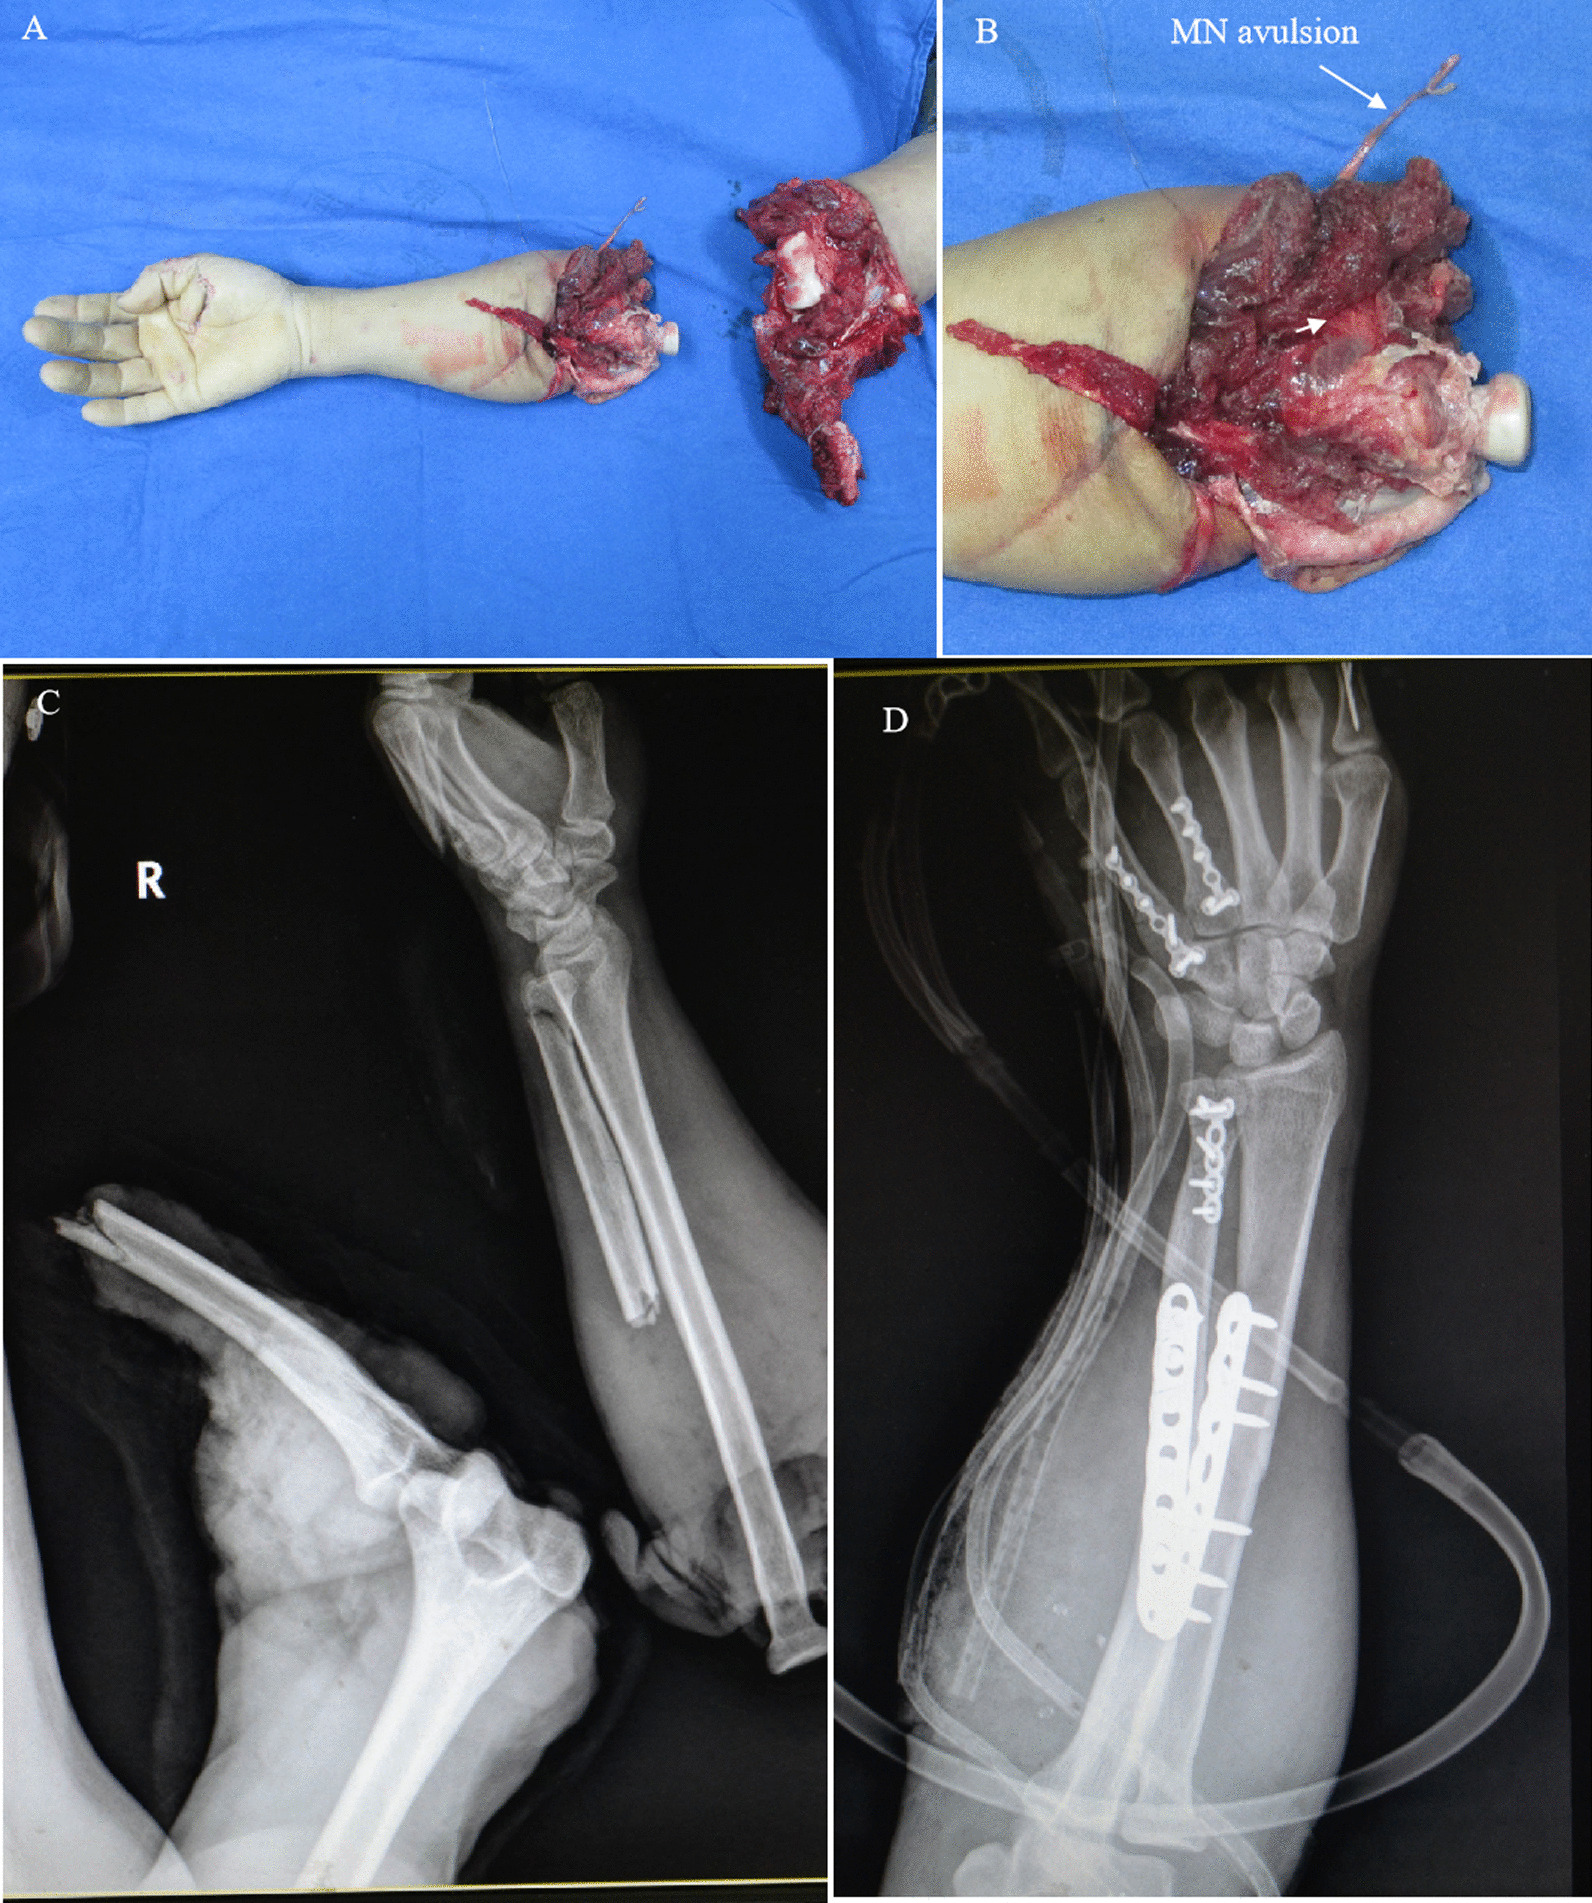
A patient (case 2) undergoing M-(M + U) repair (part 1). A, B The subject suffered avulsion amputation of the elbow joint and MN and UN in the right upper limb. C Preoperative X-ray showed that the subject had elbow joint dislocation and multiple fractures of the ulna and metacarpal bones. D Postoperative X-ray showed internal fixation of the ulna and metacarpal fractures, shortening and internal fixation of the radius, and reduction of the elbow joint
